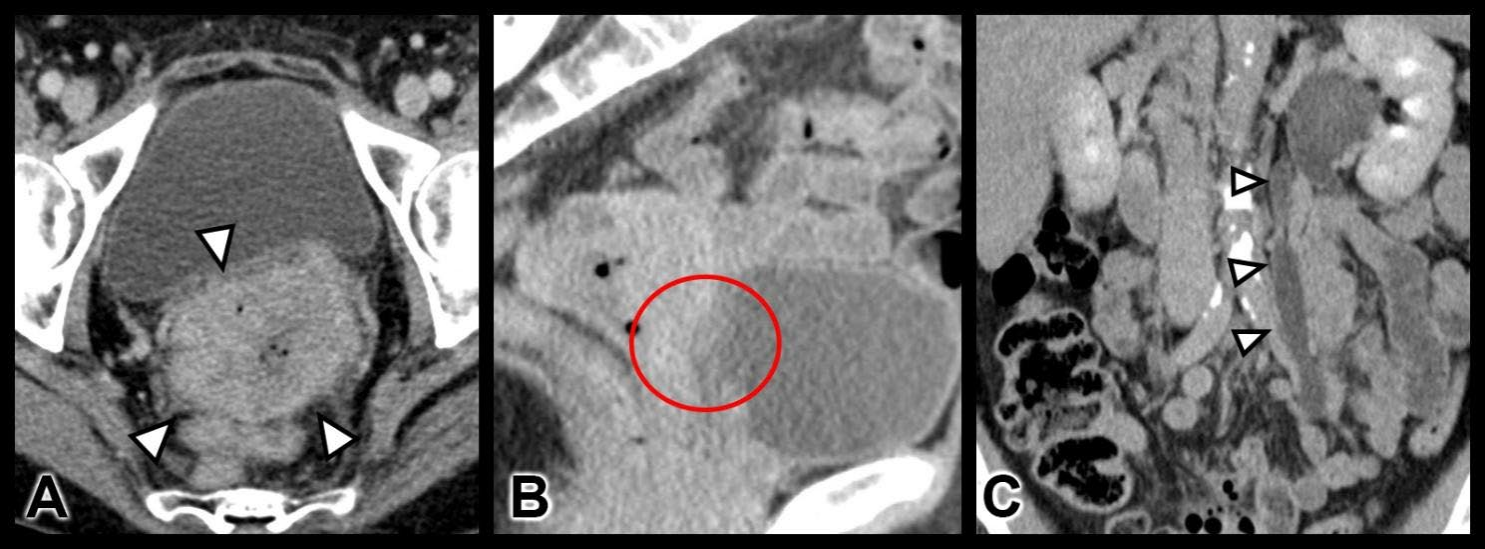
A) Axial oblique contrast-enhanced CT scan shows a large heterogeneous cervical mass abutting the urinary bladder without definite invasion. B) Sagittal contrast-enhanced CT scan shows the large exophytic cervical mass extending anteriorly into the vaginal vault. C) Coronal oblique contrast-enhanced CT scan shows left-sided stage I hydronephrosis with dilated ureter due to tumoral invasion
